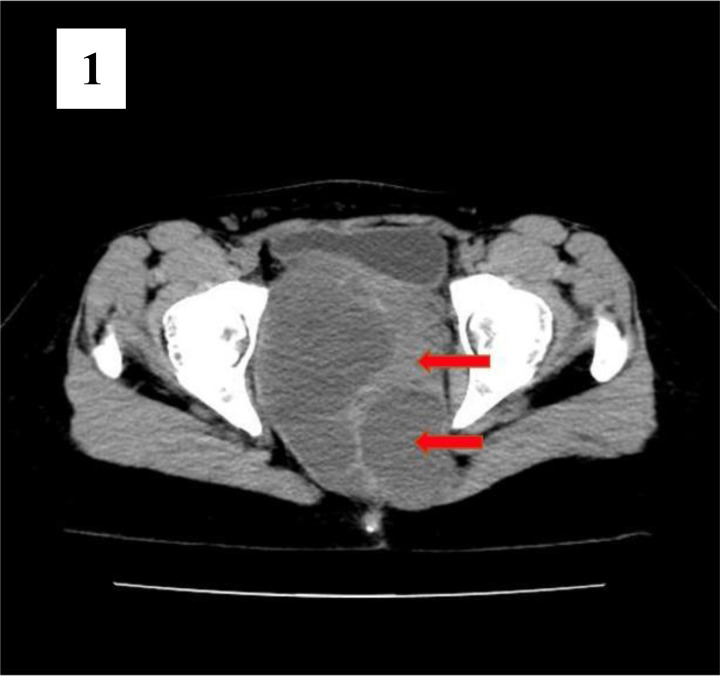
is an axial view of the non-contrast CT scan that shows both cystic and solid areas (red arrows).
